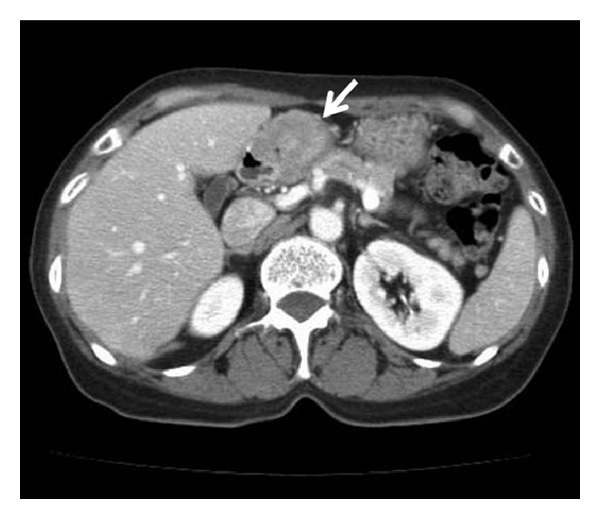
Computed tomography showed a tumor located at the antrum of the stomach (arrow), with no invasion of the primary tumor to adjacent structures and no distant metastasis.
